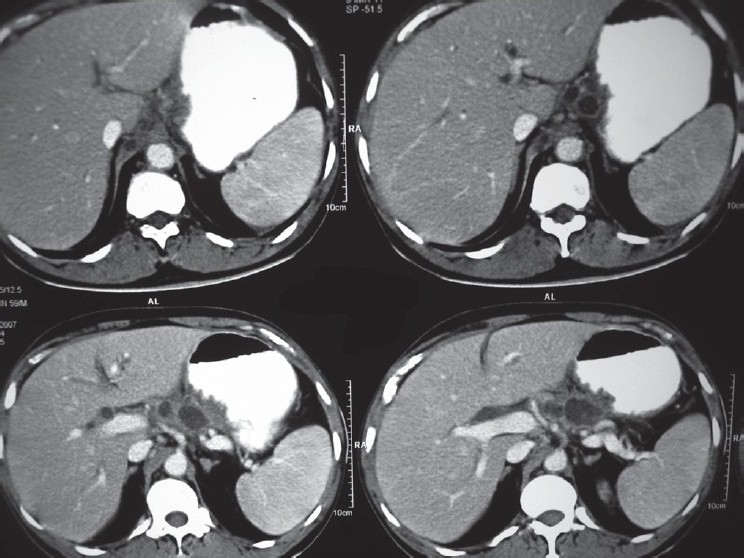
Contrast enhanced CT scan of the pancreas showing cystic lesions in the body and tail of pancreas. Splenomegaly is also noted.
